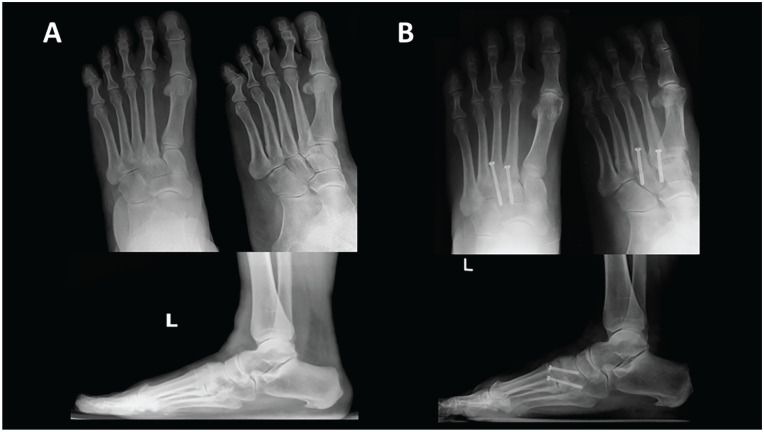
(A) Preoperative anteroposterior (AP), lateral, and oblique radiographs of an arthritis midfoot (second and third tarsometatarsal joint). (B) Sixteen-week postoperative AP, lateral and oblique radiographs from the same patient—second and third TMT joints arthrodesis using compression screws. Abbreviation: TMT, tarsometatarsal.
